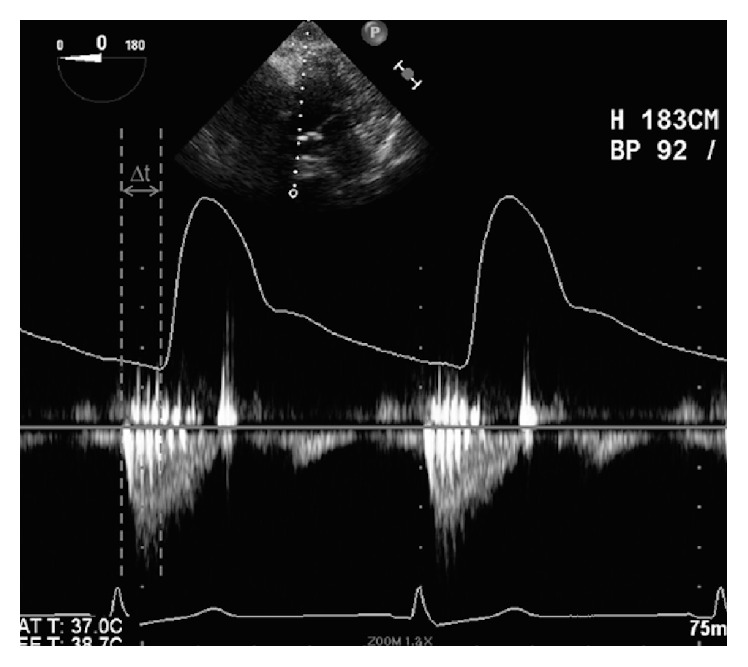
Representative image of measurements. We measured the transit time (Δ*t*) from the start of ejection on continuous wave Doppler waveform to the upstroke on the radial arterial waveform.
